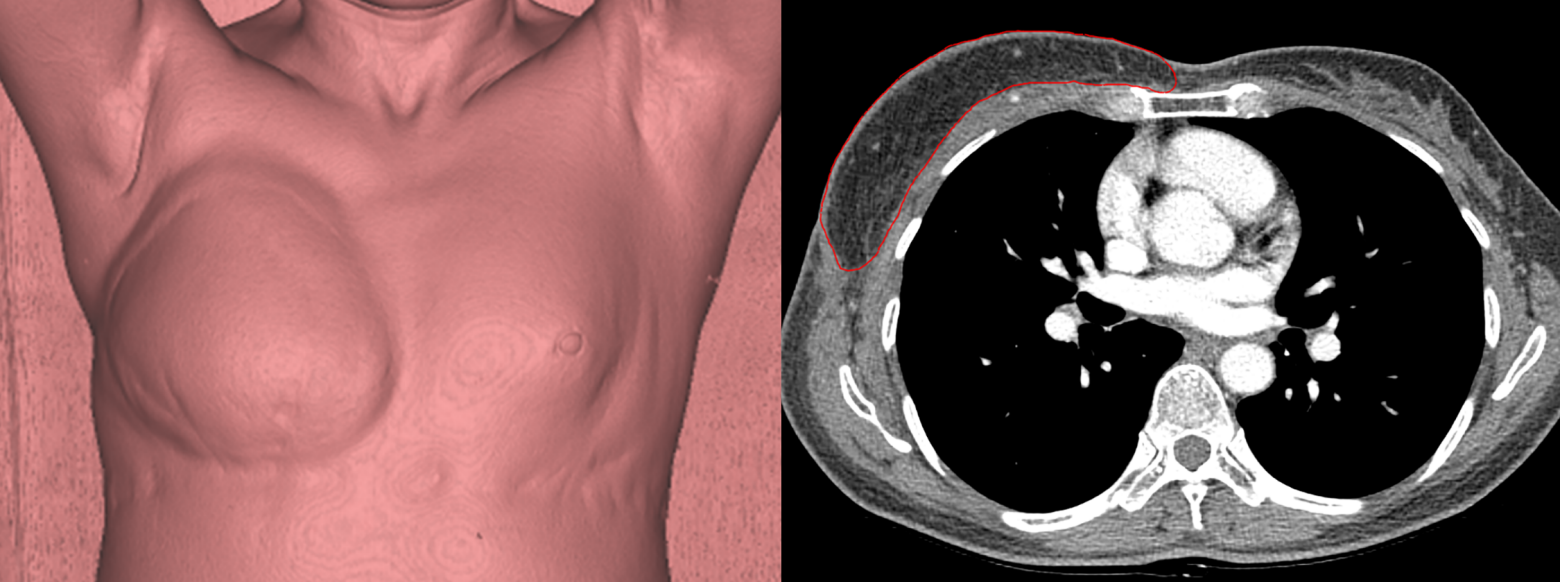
Volumetric analysis. (A) Three-dimensional reconstructed image of chest computed tomographic study. (B) Outline of abdominal flap was manually marked in each scan image.
